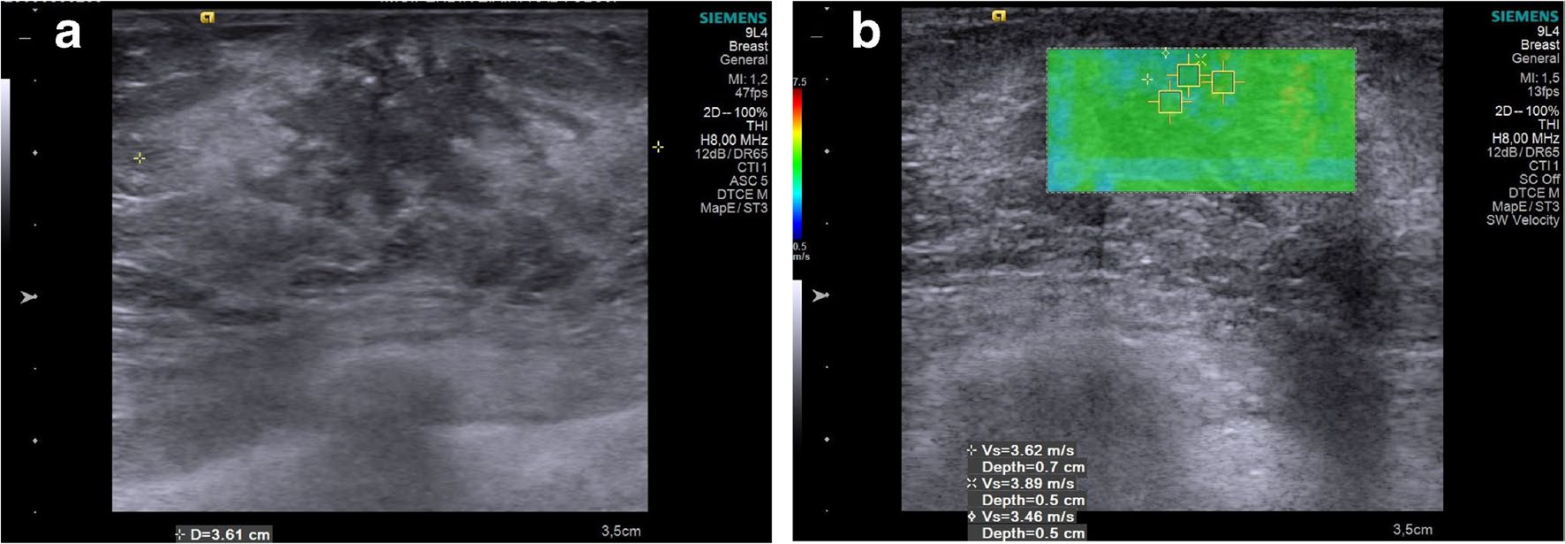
Shear-wave elastography assessment in an 8-year-old girl with a stage III breast bud who was diagnosed with premature thelarche. Grayscale axial ultrasound without (a) and with (b) shear-wave speed measurements at different locations (*squares*). Mean shear wave velocity was calculated to be 3.66 m/s (b)
